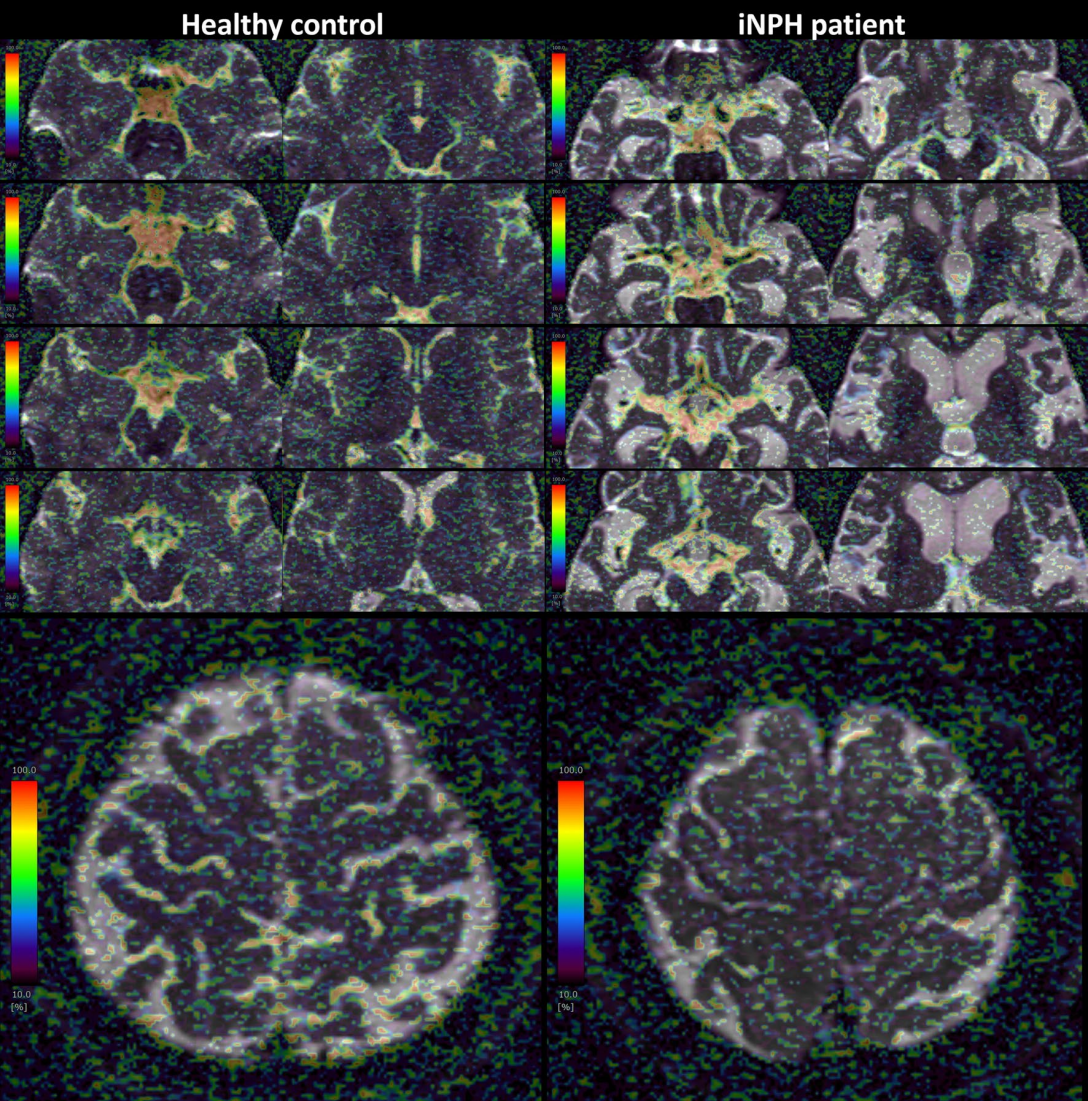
f* map (%) of IVIM MRI in the ventricles and subarachnoid spaces in a representative healthy control (left) and iNPH patient (right).
